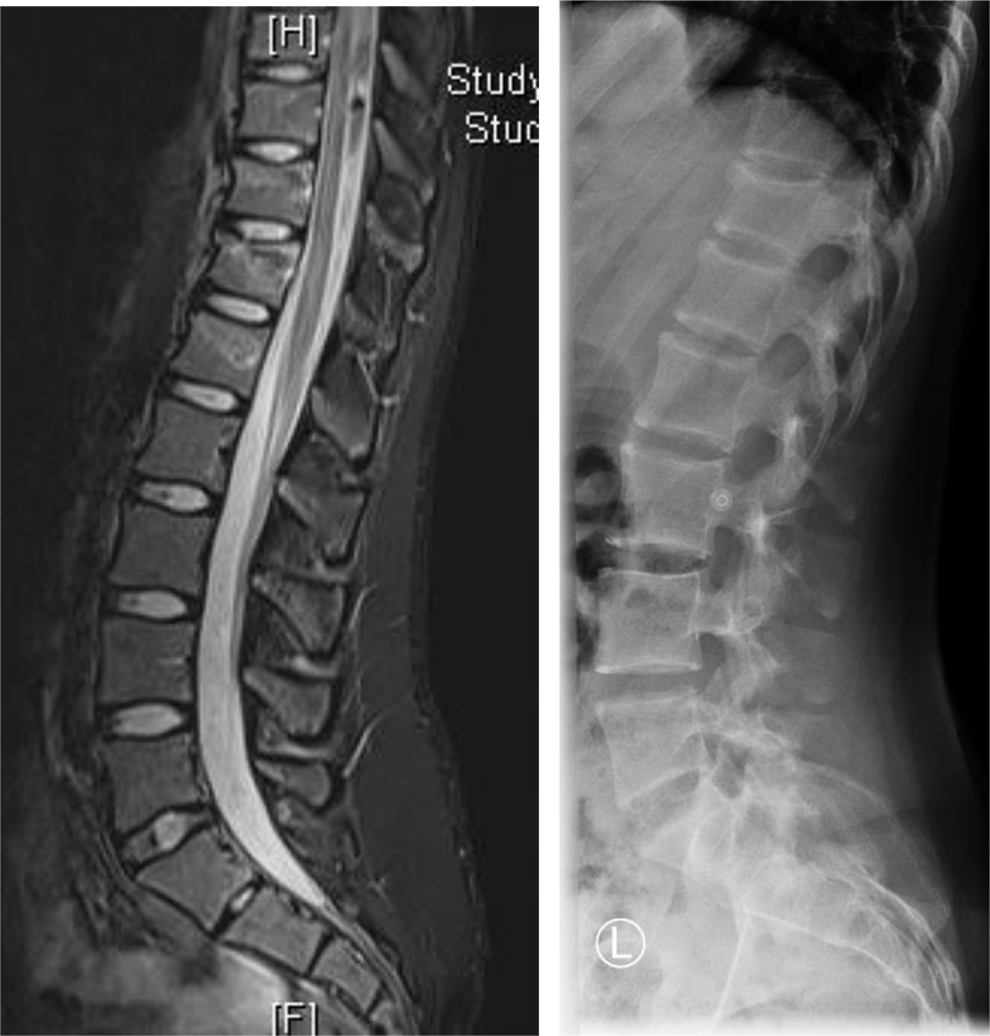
15 years, female, fall from second floor, TH 11-L1 chain injury AO type A1 L1 and A3 TH11/12, Due to her age a CT scan was not performed. Follow-up X-ray presented no further dislocation
